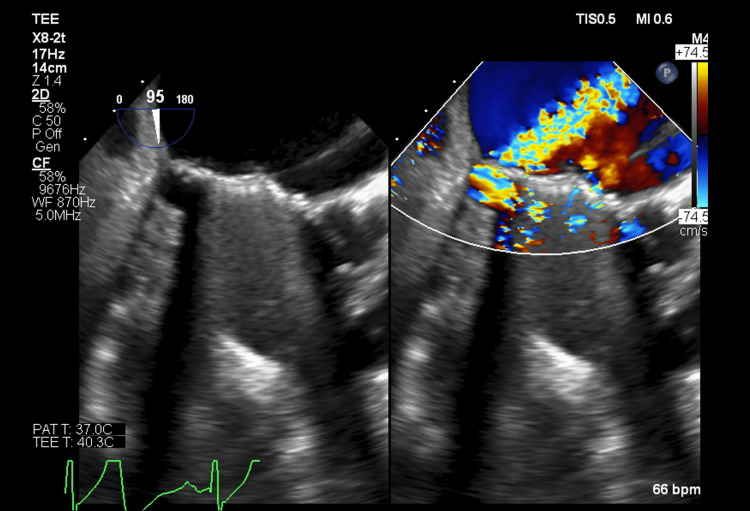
Transesophageal echocardiogram mid-esophageal two-chamber view with and without color Doppler, demonstrating paravalvular regurgitation originating from the posterior aspect of the mitral valve prosthesis.
